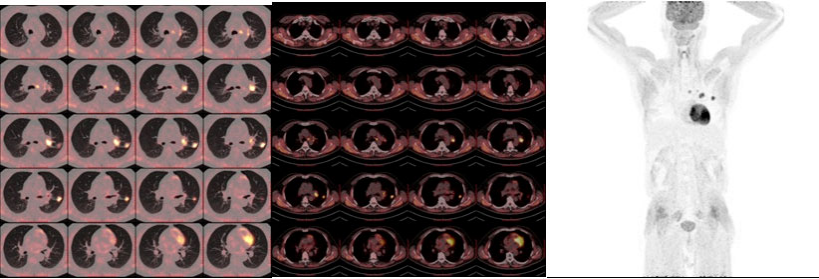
PET-CT at baseline 1 month before dabrafenib/trametinib treatment. PET-CT scan of the chest at baseline prior to dabrafenib plus trametinib therapy demonstrated a nodule in the upper left lung with elevated uptake of 18F-fluorodeoxyglucose (FDG), accompanied by enlargement of multiple lymph nodes of the left lung hilum as well as mediastinum (4L, 5, 6). No other lesions with elevated uptake of 18F-FDG were found.
